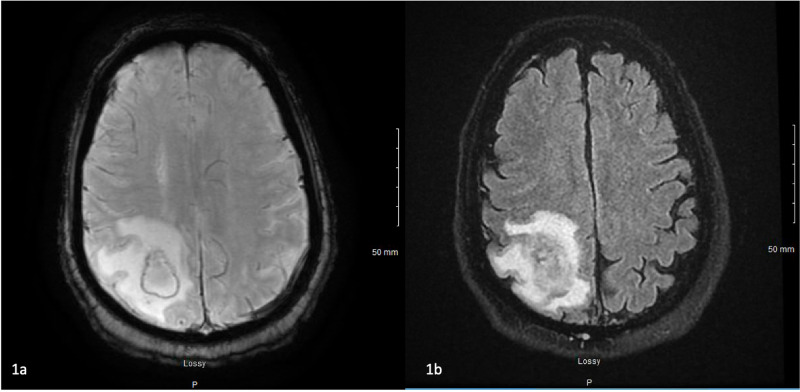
Initial MRI head showing 3.1 x 2.3 cm ring-enhancing lesion in the right parietal lobe Figure 1a: axial susceptibility-weighted imaging (SWI), Figure 1b: axial fluid-attenuated inversion-recovery (FLAIR) fat-suppressed (FS) imaging
